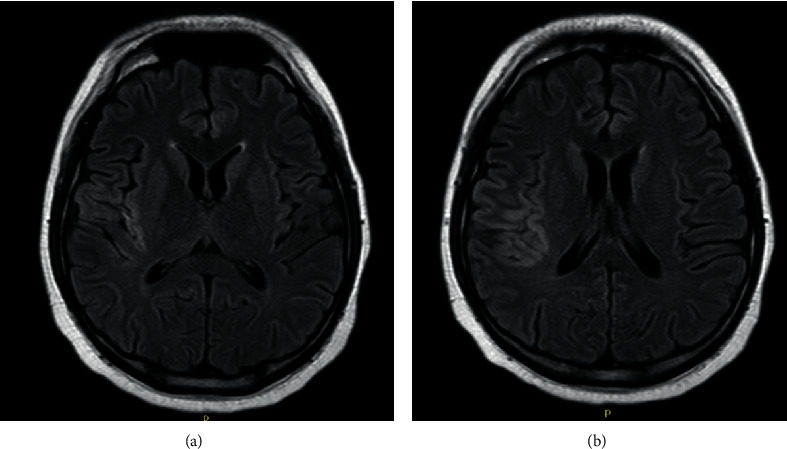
FLAIR: hyperintensity over right temporal lobe and insular region.
